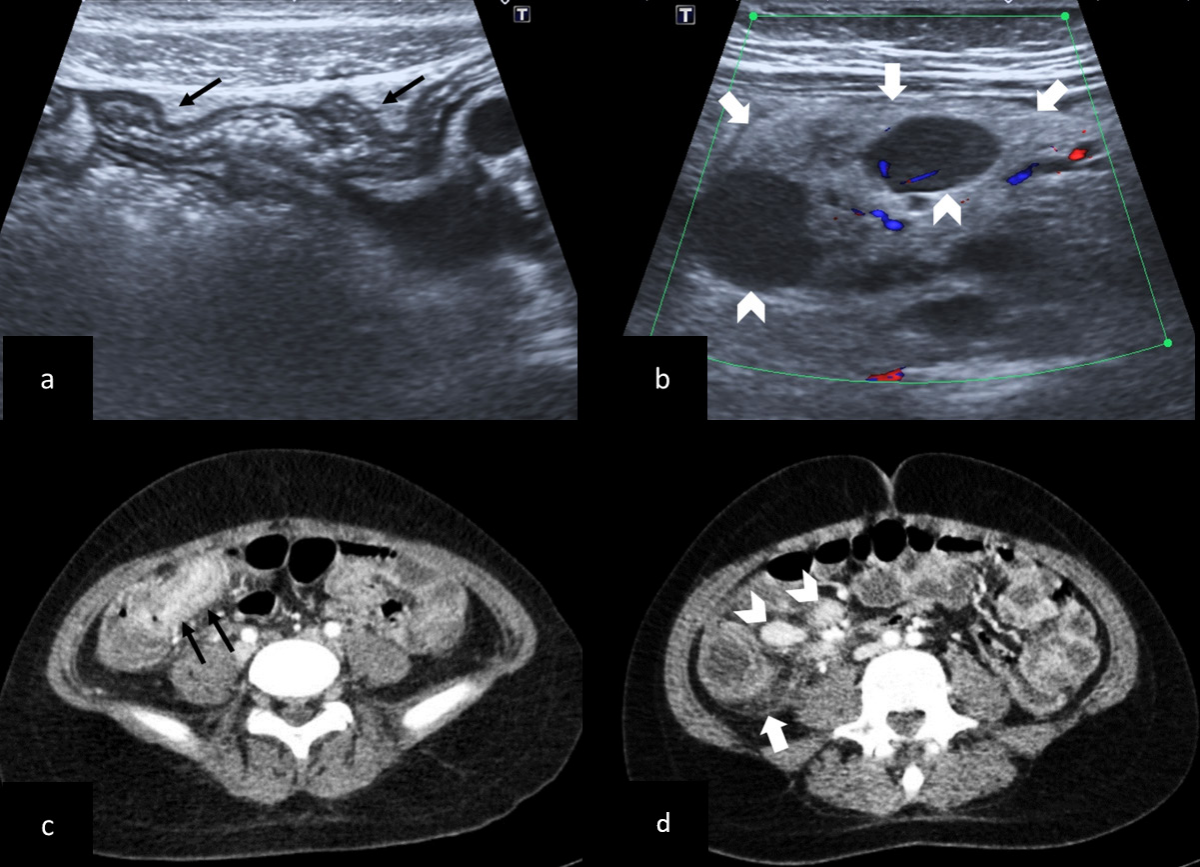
Imaging findings of an inflammation of the right iliac fossa in a 12-year-old girl who presented with fever, abdominal pain, diarrhea, and vomiting. (a–d) Ultrasonography (a, b) and axial CT images (c, d) show thickening of the terminal ileum wall (black arrows in a, c) and hyperechoic-thickened mesenteric fat (white arrows in b, d) around multiple mesenteric lymph nodes (arrow heads in b, d).
